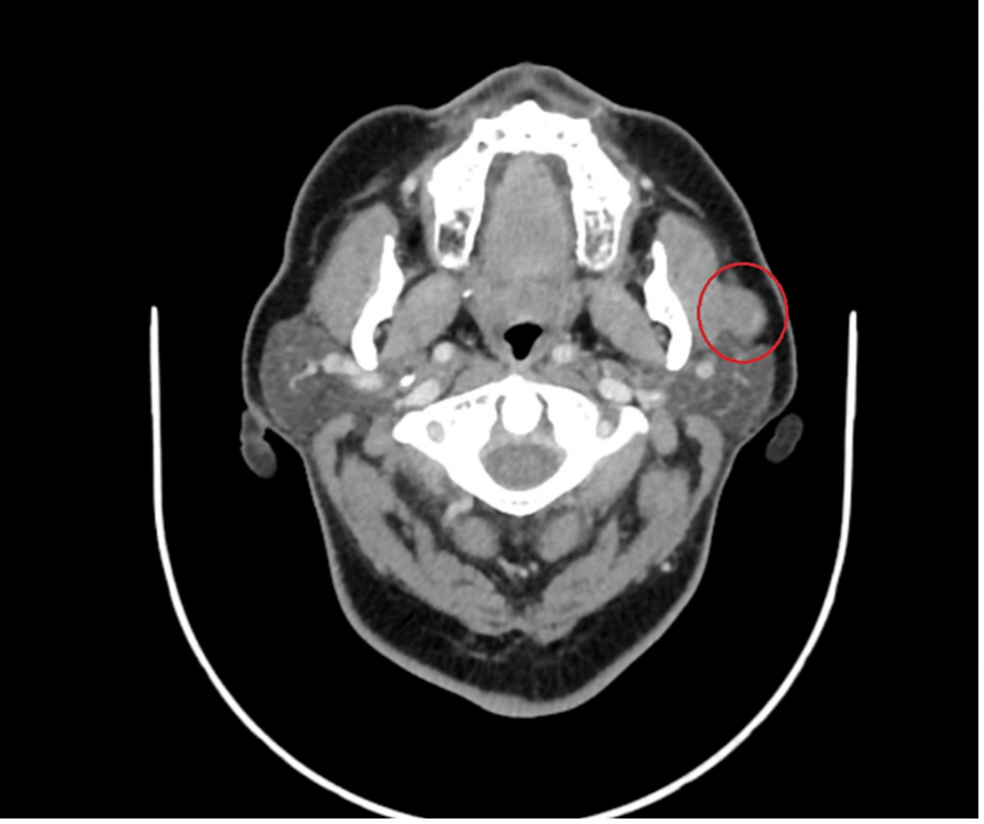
CT head and neck (axial section) An enlarged, non-enhanced oval shaped left mandibular mass located superficial to and lying on the left masseter muscle measuring 1.2 cm with foci of calcification; minimal peripheral enhancing components.
